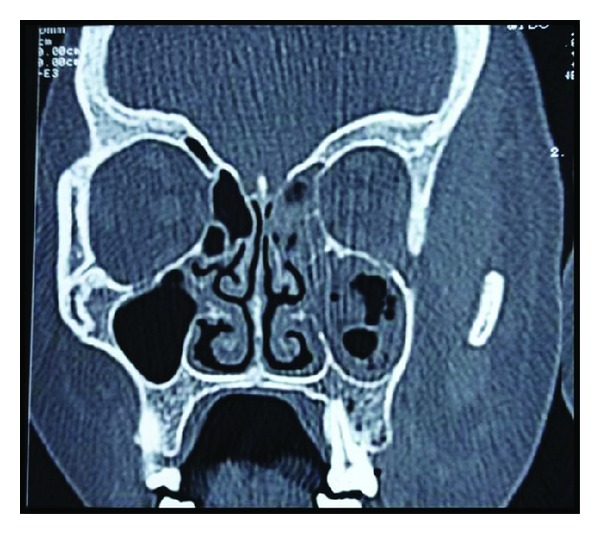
Coronal view of CT showing involvement of left maxillary sinus, nasal conchae, and ethmoidal sinus extending up to frontal sinus.
